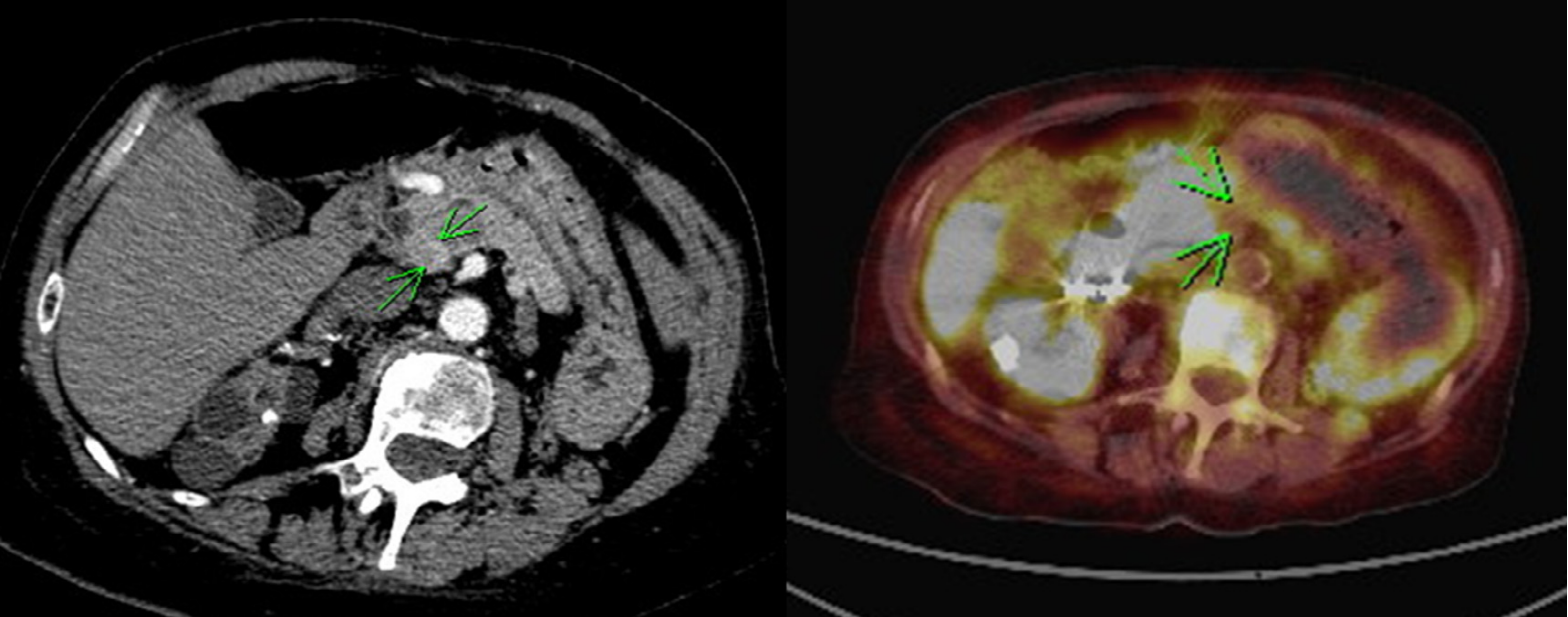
Abdominal computed tomography scan (CT) following a pancreatic protocol (left) and Gallium DOTATATE positron emission tomography/computed tomography scan (PET/CT) (right). Green arrows indicate a 1.5‐cm lesion between the head and uncinate process consistent with a neuroendocrine tumor.
